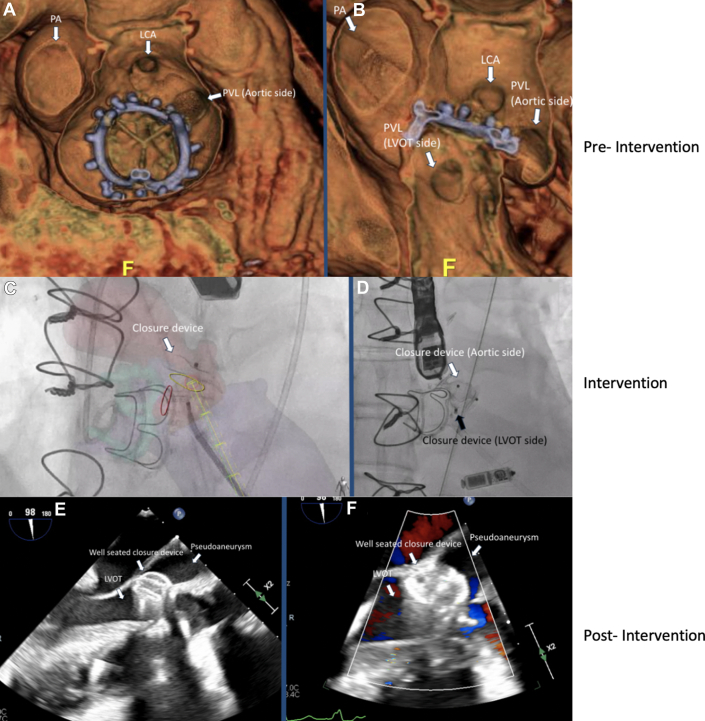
Workup and Treatment of a Large Aortic Paravalvular Leak and Pseudoaneurysm (A and B) Preintervention CT shows the origination of the PVL and the entry into the LVOT. (C) CT-fluoroscopy fusion imaging used to guide the procedure to help close the LVOT side from the transapical approach, and (D) cineangiography showing well-seated closure of the aortic and LVOT side. (E) Postclosure transesophageal echocardiography shows vascular plug preventing flow from the pseudoaneurysm into the LVOT, and (F) color Doppler shows no flow from the pseudoaneurysm into the LVOT. CT = computed tomography; LCA = left main coronary artery; LVOT = left ventricular outflow tract; PA = pseudoaneurysm; PVL = paravalvular leak.
